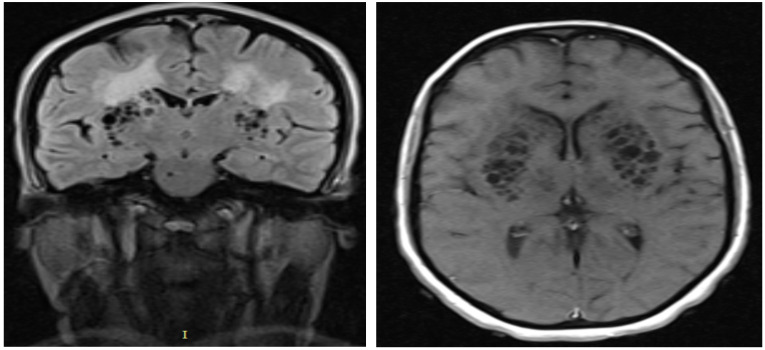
a) Coronal FLAIR MRI showing bilateral multiple cystic lesions in basal ganglia region having CSF-like content with increased signal intensity of the surrounding brain parenchyma; b) Axial T1 weighted MRI after gadolinium injection, showing bilateral non-enhancing multiple hyporintense cystic lesions in basal ganglia region
